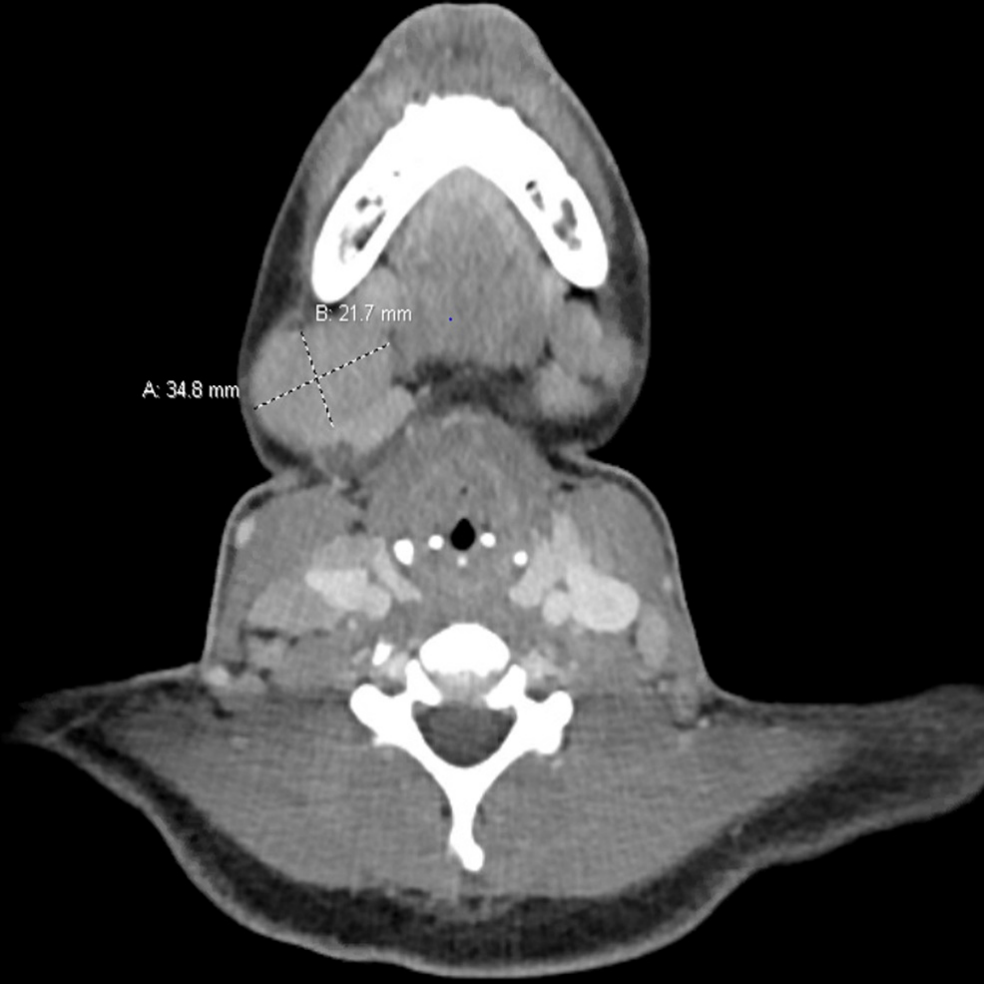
CT scan of neck with IV contrast showing bilateral cervical lymphadenopathy with the largest one measuring approximately 2.2 x 3.5 cm lymph node in the right submandibular region
